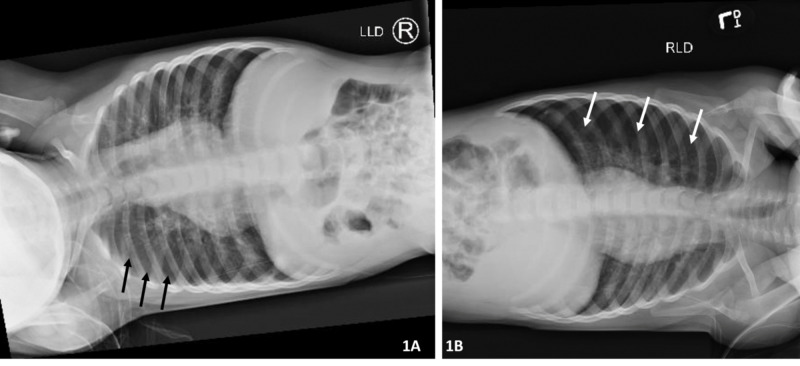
A) Left lateral decubitus X-ray on day four demonstrated air trapping in the left lung without physiological lung collapse. Black arrows outline the lateral aspect of the lung. B) Right lateral decubitus physiological lung collapse with left side pneumothorax. White arrows outline the lateral aspect of the lung
